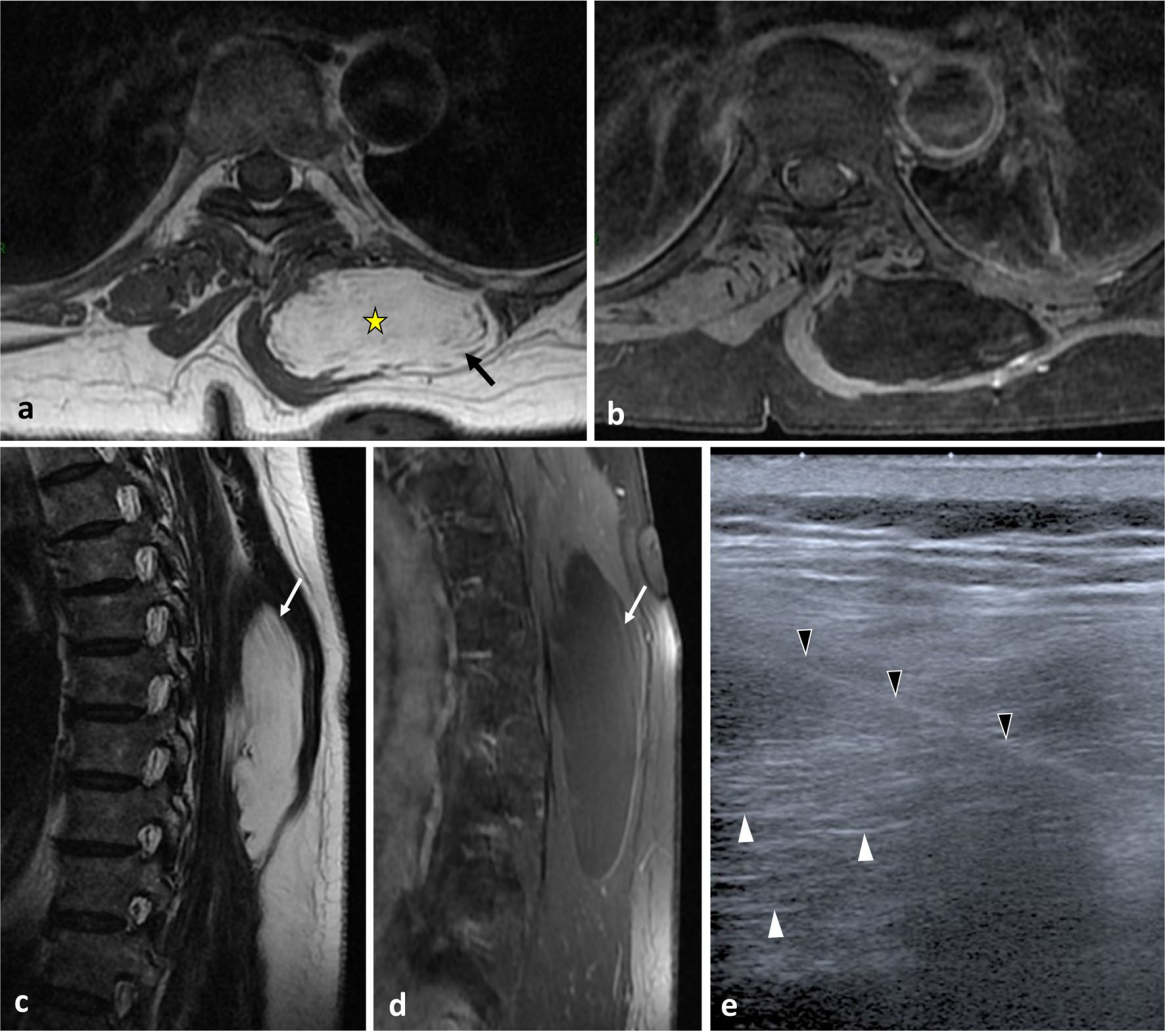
Intramuscular lipoma* in the left erector spinae muscle in a 60-year-old male with soft, painless and non-inflammatory paraspinal tumefaction. MRI demonstrates the typical features of intramuscular lipoma: high intensity on T1WI (a), saturation on fat-saturated T1WI (b), high intensity onT2WI (c) and absence of enhancement on contrast-enhanced fat saturation T1WI (d). Streaky structures correspond to entrapped muscle fibres (arrows). US-guided biopsy (e, black arrowheads) shows a hyperechoic mass with thin transversal lines (white arrowheads). Pathological report demonstrates negative detection for MDM2 supporting classification as lipoma instead of atypical lipomatous tumour. The yellow star shows the epicentre of the mass
